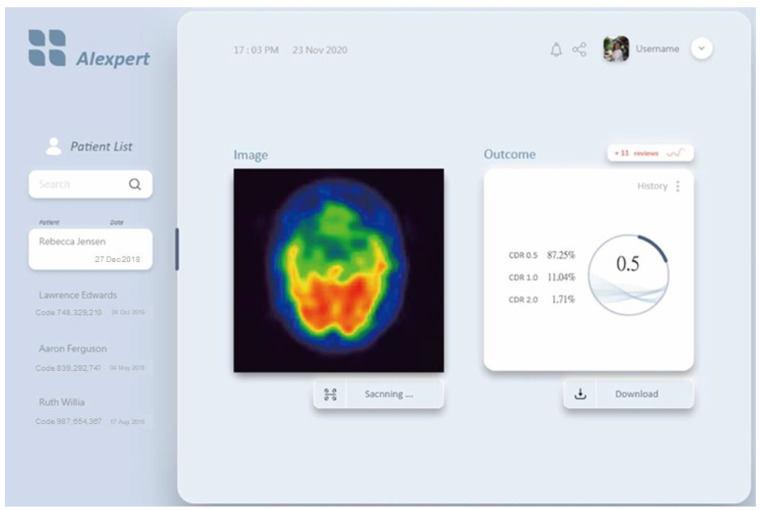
AI expert system based on the ResNet model. Physicians can import the SPECT image (left part), and the AI expert system will show the outcome (here, of 0.5) (right part) to help them make decisions on disease classification.
